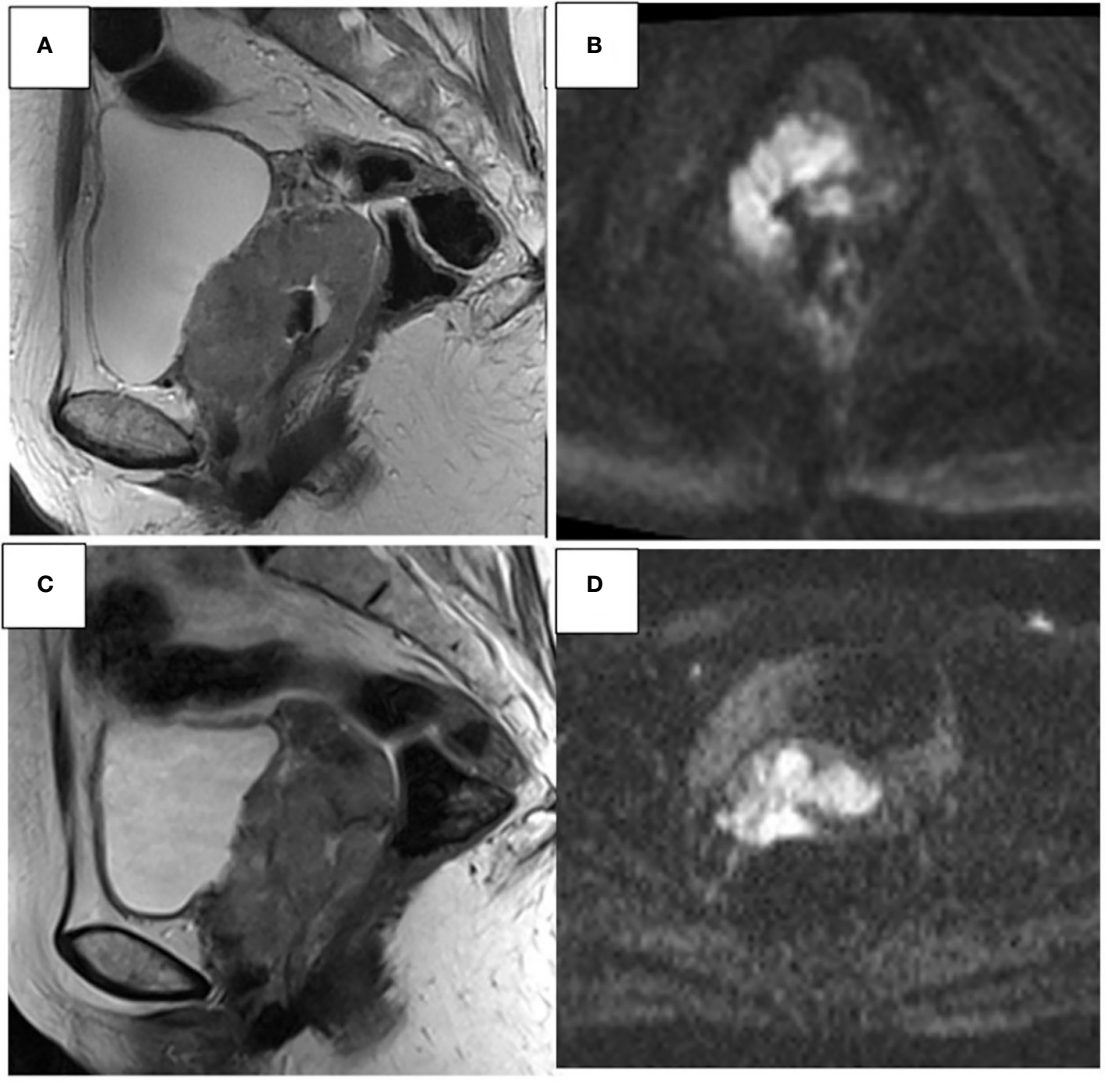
45- year-old woman. Sagittal T2W and axial DWI MR images show an invasive CC with parametrial invasion and extension of the upper 2/3 of the vagina (A, B). Stable disease (C, D) after CCRT, CC is changed in morphology and size; however, infiltration of the vaginal fornix, upper 2/3 of the vagina, and both parameters remain.
